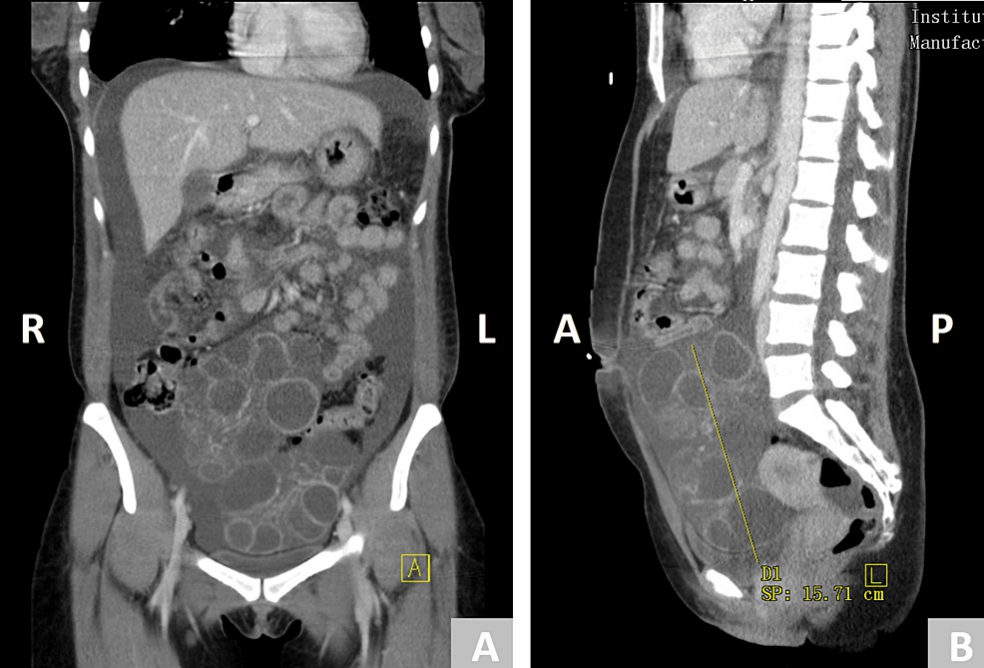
Coronal (2A) and sagittal (2B) views of CT imaging of the abdomen and pelvis demonstrate ascites with a large collection of ovarian follicles.
